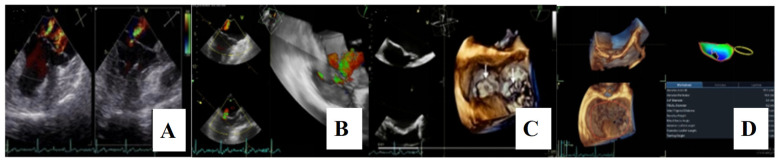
(A,B)—Transoesophageal echocardiography (TEE) showing severe mitral regurgitation. (C,D)—TEE 3D view, displaying bileaflet prolapse and mitral annular enlargement. Mitral valve apparatus geometry and mitral valve dynamics were evaluated using EchoPAC software version BT12.
